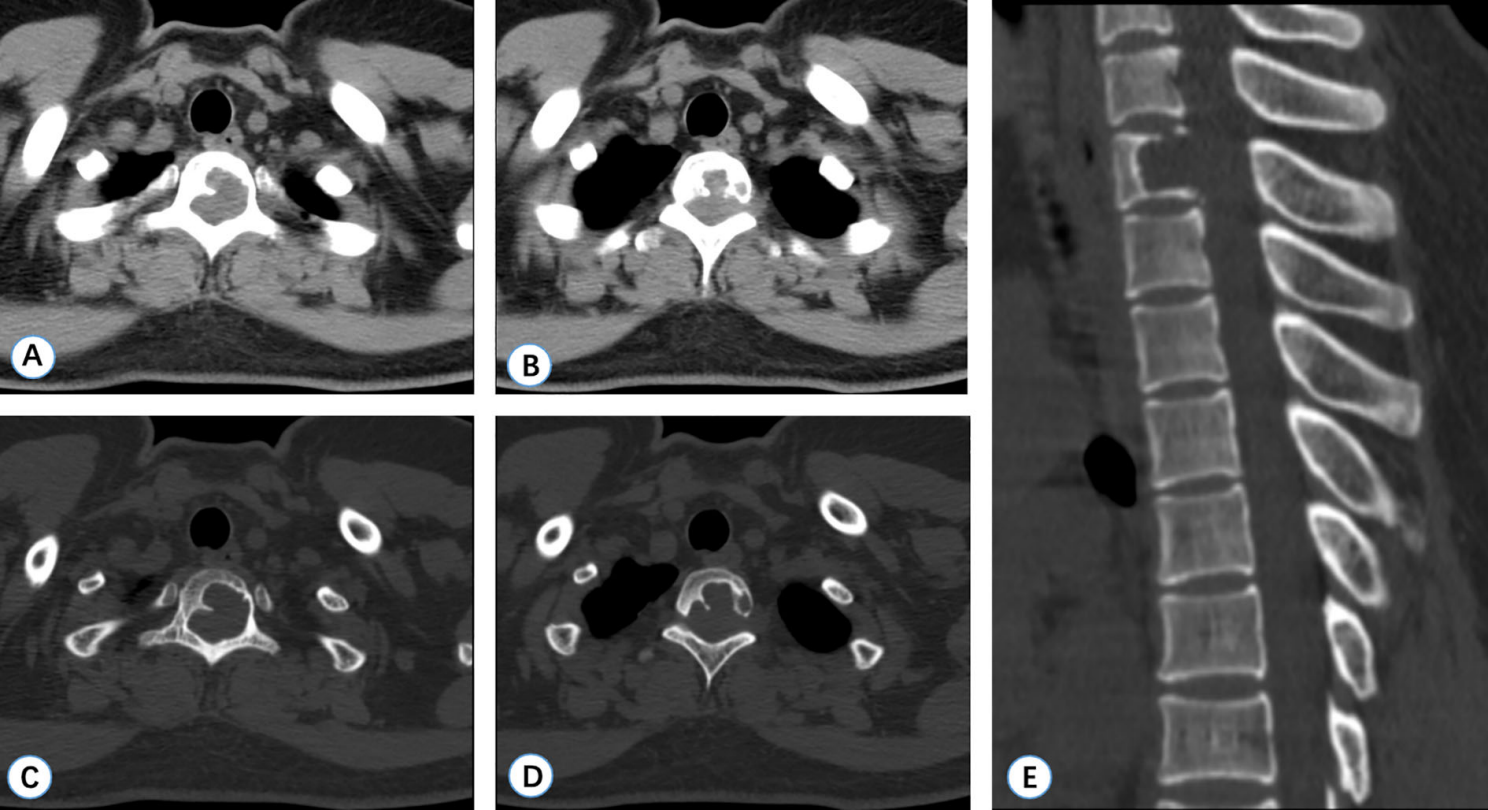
CT manifestations of Case 1 (T2 vertebra). (A–D) Axial CT scans reveal osteolytic bony destruction of the T2 vertebra with intraspinal extension. (E) A sagittal CT scan demonstrates a bony defect in the T2 body.
